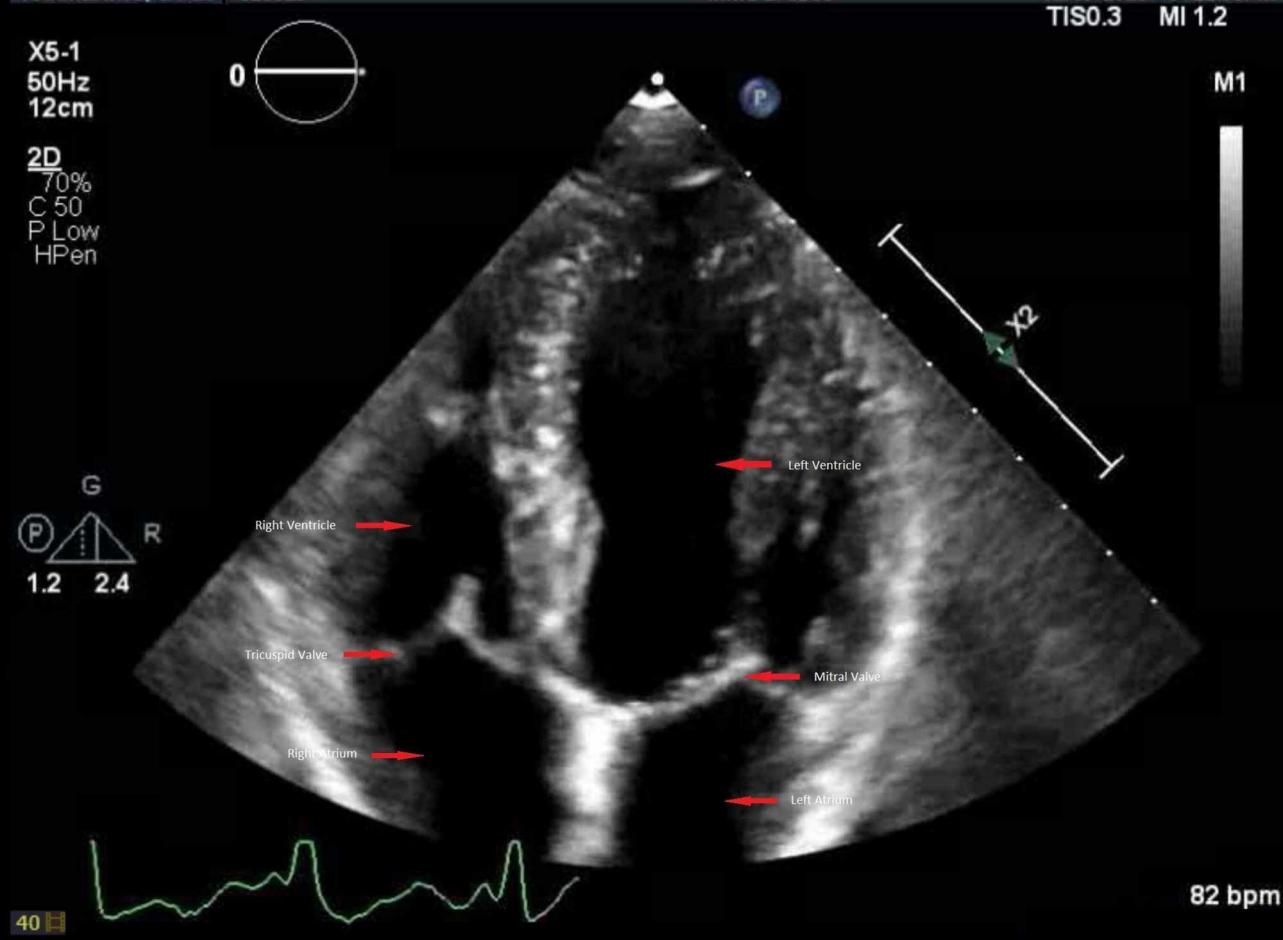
Echocardiogram of the patient with labelled chambers and valves (red arrows), which was found to be within the normal limits.
